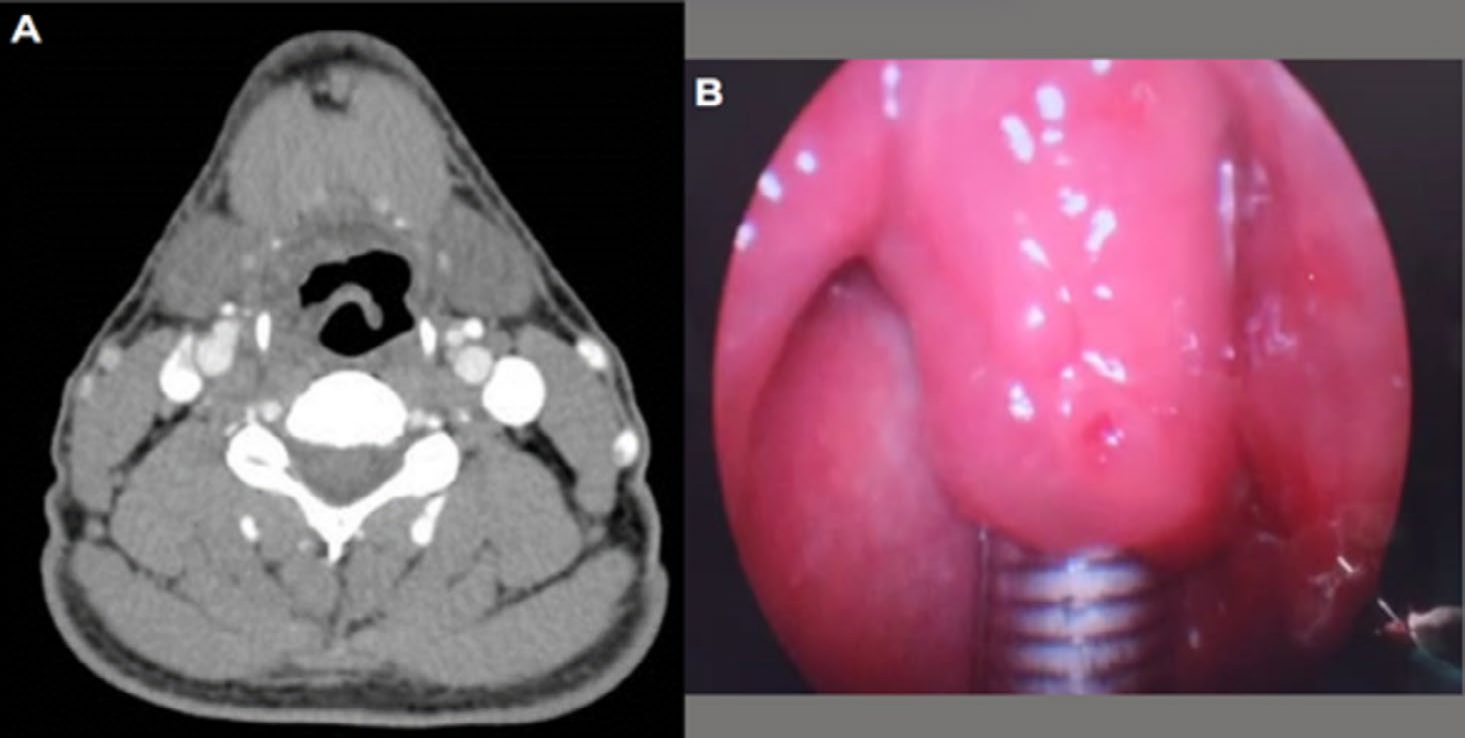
Follow‐up CT scans (A) and direct laryngoscopy (B), demonstrating absence of lesion without any curative intervention.
